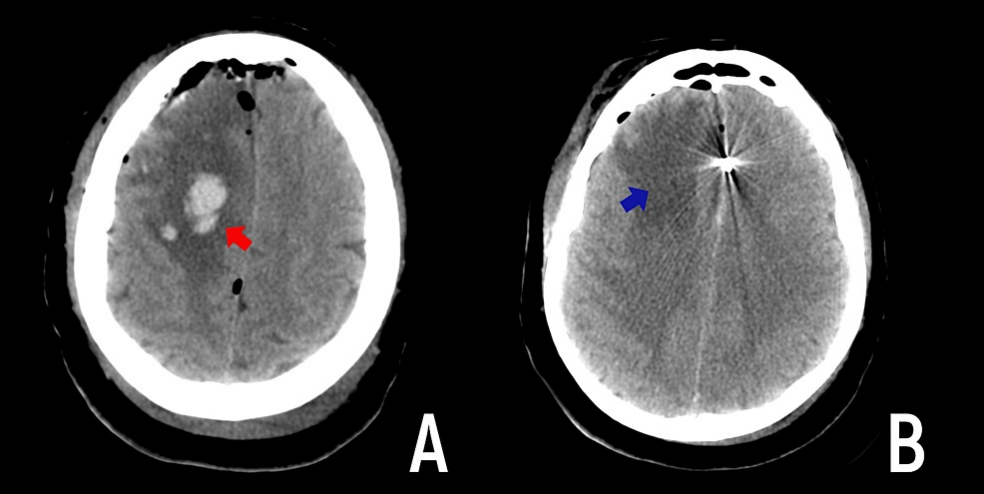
Clinical observation-hemorrhagic complication in case number 2 CT scan of the head on the first day after clipping of the aneurysm of the right pericallosal artery through the interhemispheric approach. A pronounced swelling of the right frontal lobe with hemorrhagic impregnation (red arrow) is shown. B - Venous ischemia of the right frontal lobe (blue arrow).
